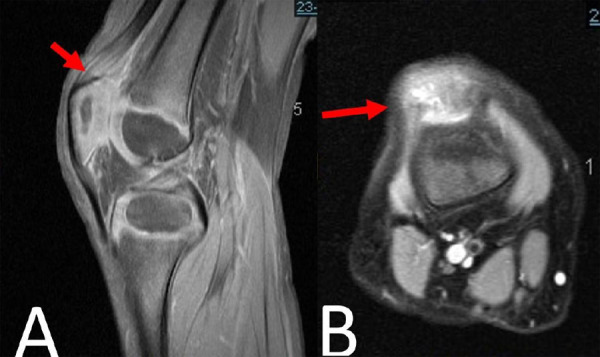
MRI in the coronal (A) and axial (B) section showing the high signal intensity (arrow) of the right knee highlighting the partial quadriceps tear just proximal to its patellar insertion.
